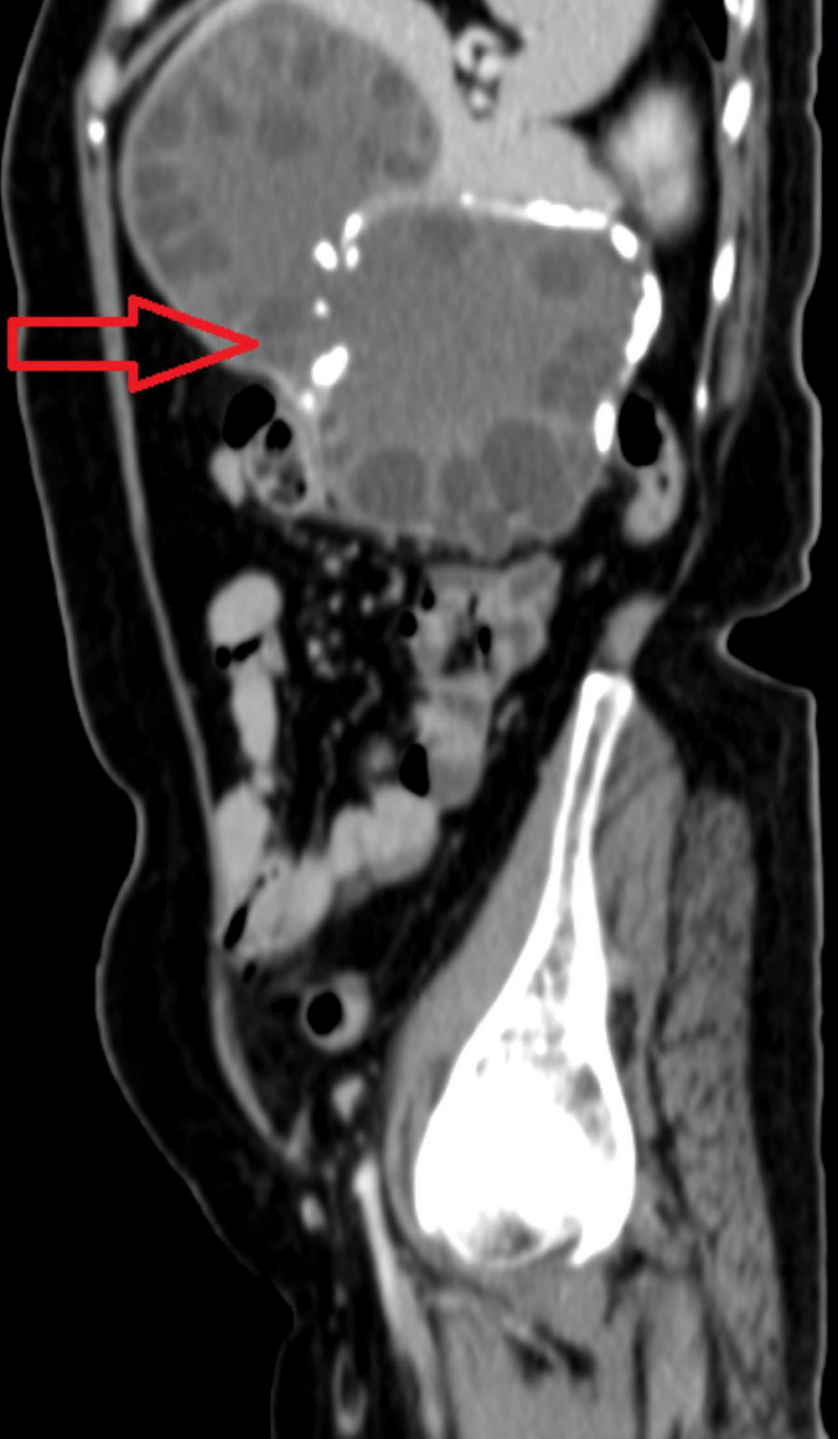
CT scan sagittal window showing multiloculated hypodense cyst multiple peripherally located daughter cysts. Wall of the lesion was partially calcified. The lesion measured 14 × 7.7 × 13.2cm.
